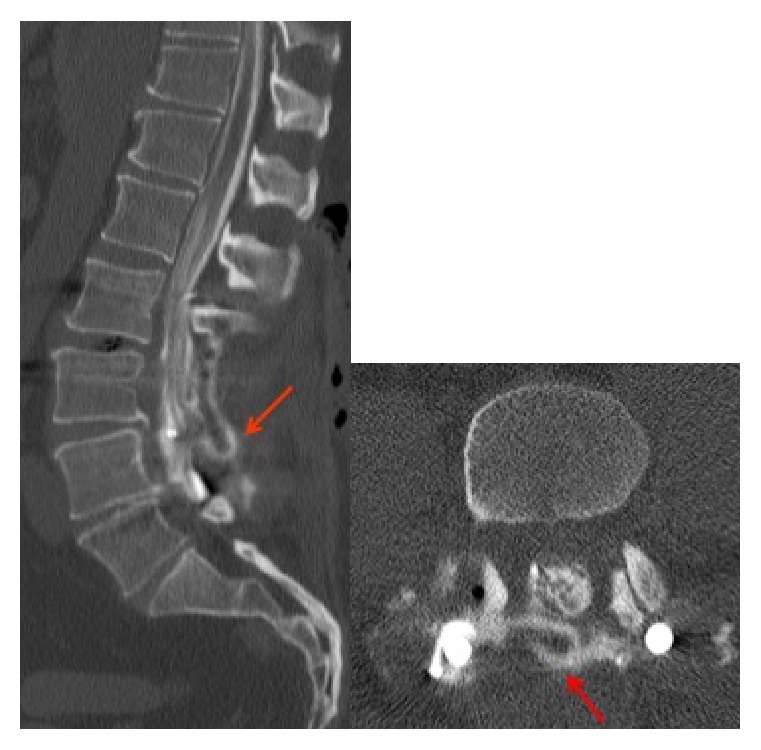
Sagittal and axial CT lumbar myelogram images show a focal CSF leak from the posterolateral aspect of the thecal sac at L3-L4 level, with iodinated contrast accumulating posterior to the thecal sac (red arrows).
